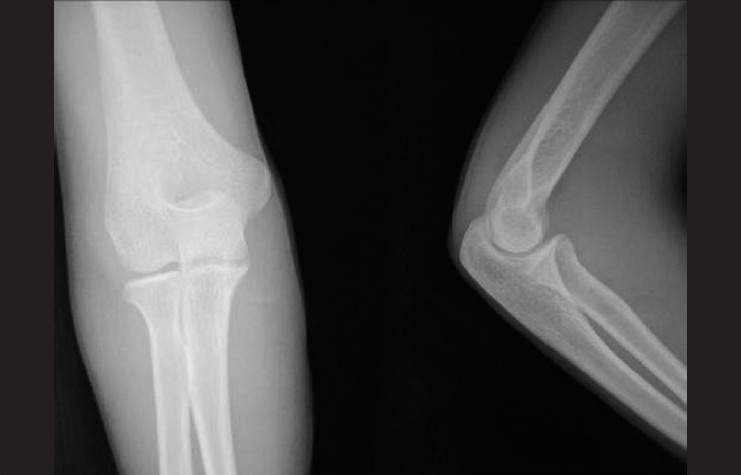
Postreduction radiographs of the elbow showing the radial head in reduced position
